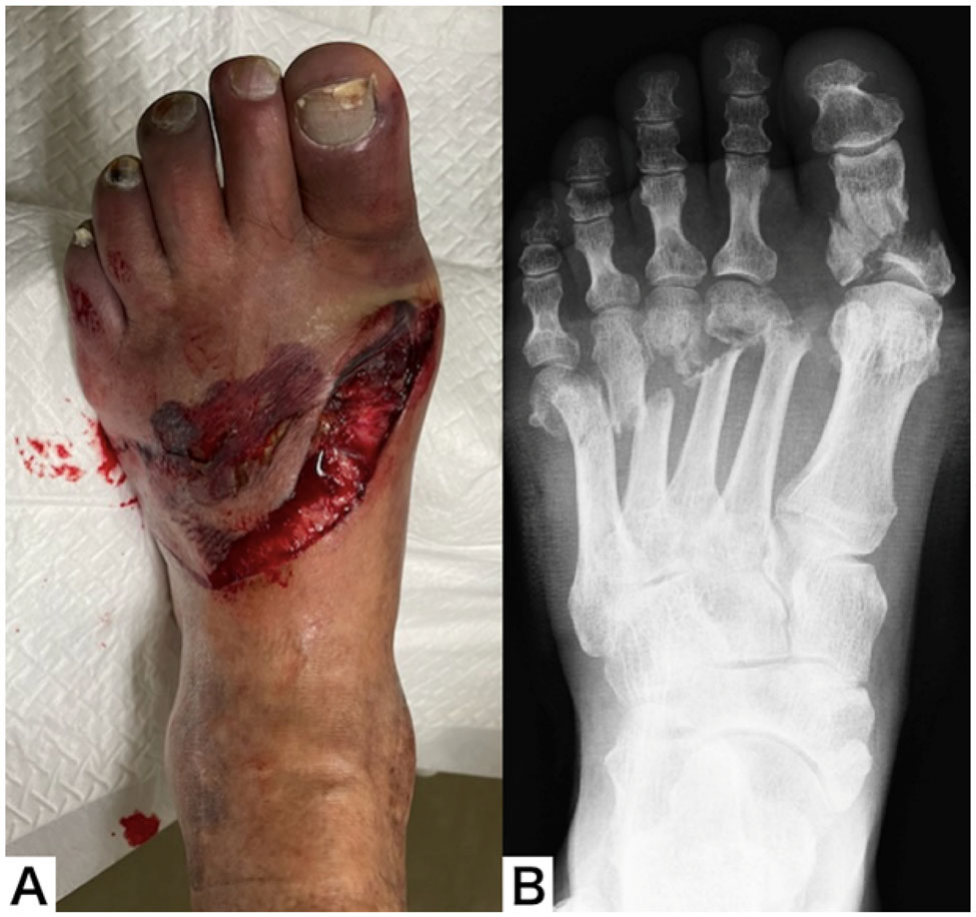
Appearance (A) and plain radiograph (B) of the left foot on Arrival at the trauma center.
